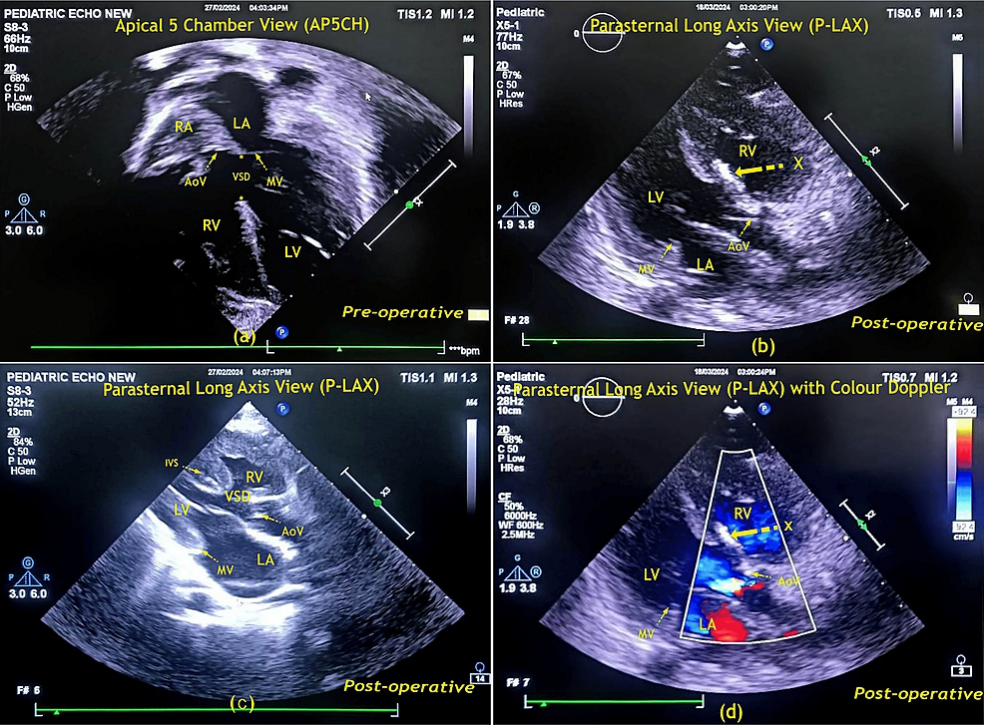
2D trans-thoracic echocardiography findings. (a) pre-operative view, (b-d) postoperative view RA: right atrium; LA: left atrium; AoV: aortic valve; MV: mitral valve; RV: right ventricle; LV: left ventricle; IVS: inter-ventricular septum; VSD: ventricular septal defect; X: VSD closed with glutaraldehyde-treated autologous pericardial patch. Image credits: Dr. Mahesh H. Bhatt/Mr. Naresh J. Fumakiya
